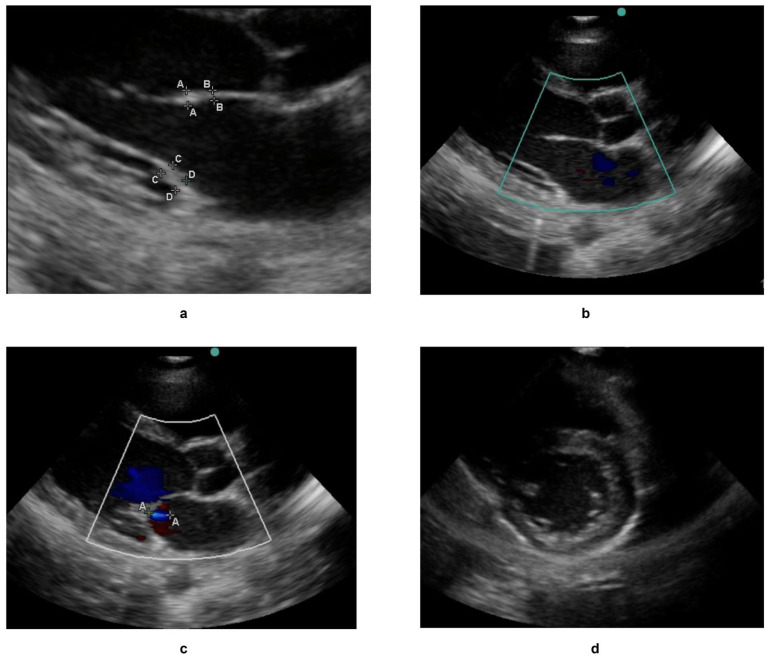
A sample of echocardiograms used as part of the labelled dataset, with the corresponding labels of all four images shown in Table 1. (a–c) are echocardiograms with a parasternal long axis view while (d) is an echo with a parasternal short axis view. The thickness state, RHD condition if any, and the severity of RHD condition if present have also been shown in Table 1. The uppercase letters, such as A–A or B–B denote standardized measurement points for structures such as valve thickness.
